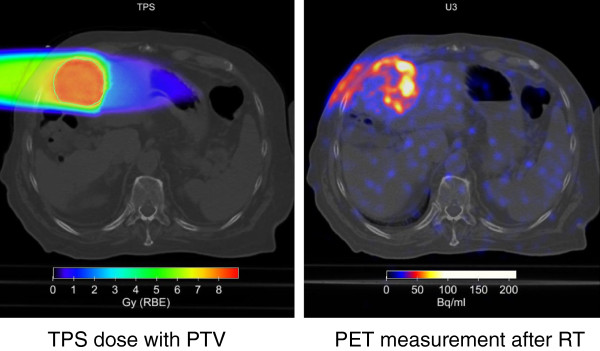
Measurement of β^+^-activity at a dedicated PET/CT scanner after application of a dose of 10 Gy(RBE). Calculation of dose distribution with Syngo^®^ RT planning (TPS).
